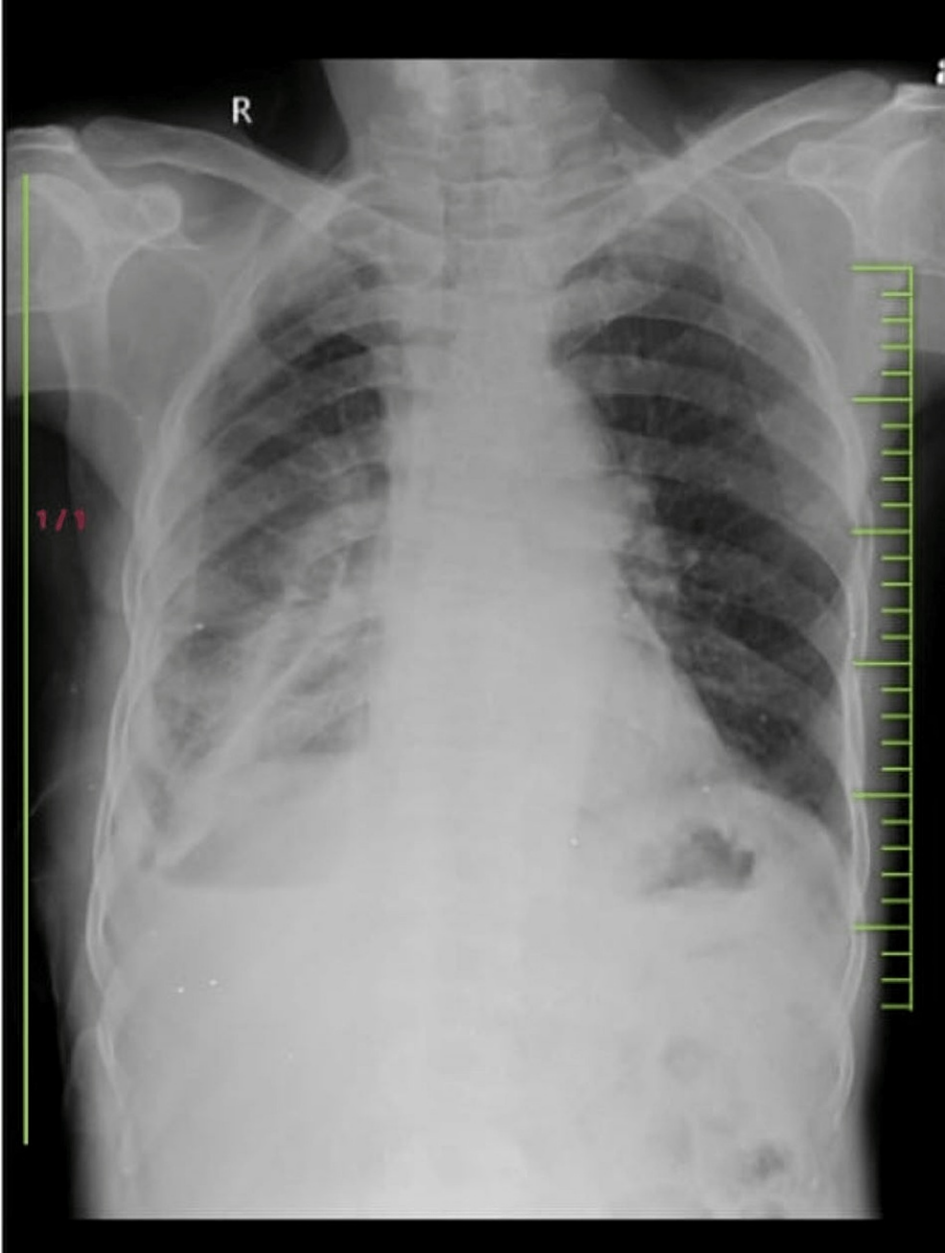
Chest X-ray of the patient (posteroanterior view). Heterogenous opacification can be noted in the right lower lobe of the lung with air bronchogram. Right lower lobe consolidation can be noted along with loculated right-sided pleural effusion.
